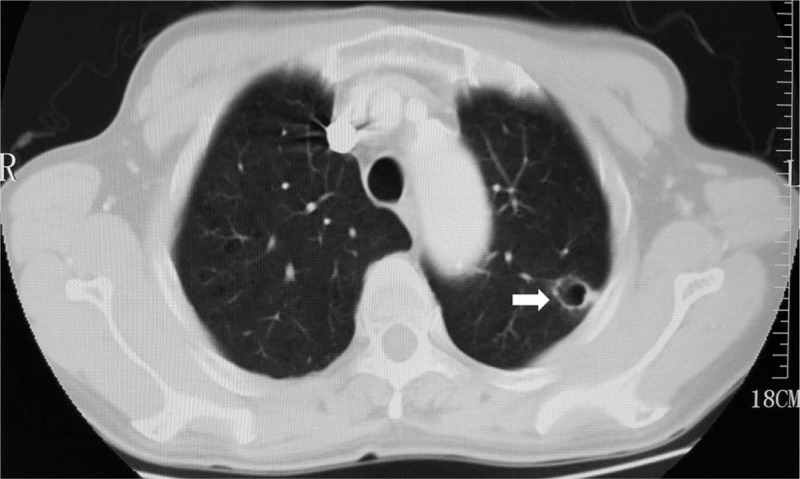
Lung-window computed tomographic image of the patient indicated an isolated thin-walled cystic lesion in left upper lobe (arrow) on November 11^th^, 2015.
